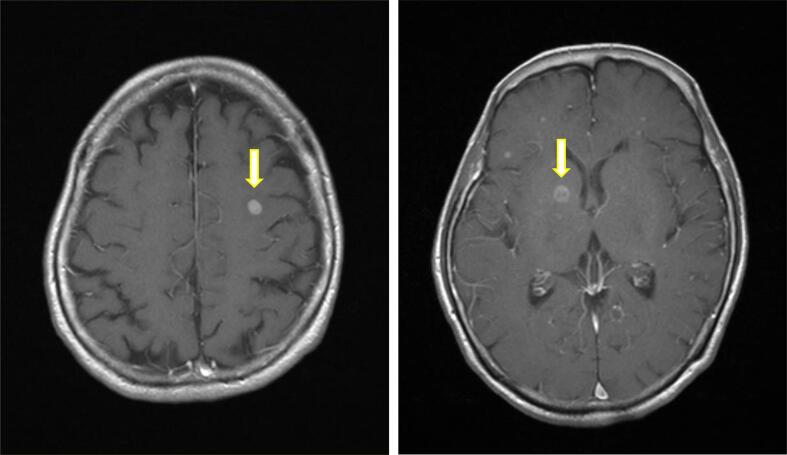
Multiple brain metastases in the white matter of the bilateral cerebral hemispheres (arrow, left and right), the right basal ganglia, and the left dorsal midbrain in Case 1 (axial post-contrast T1-weighted brain MRI).
